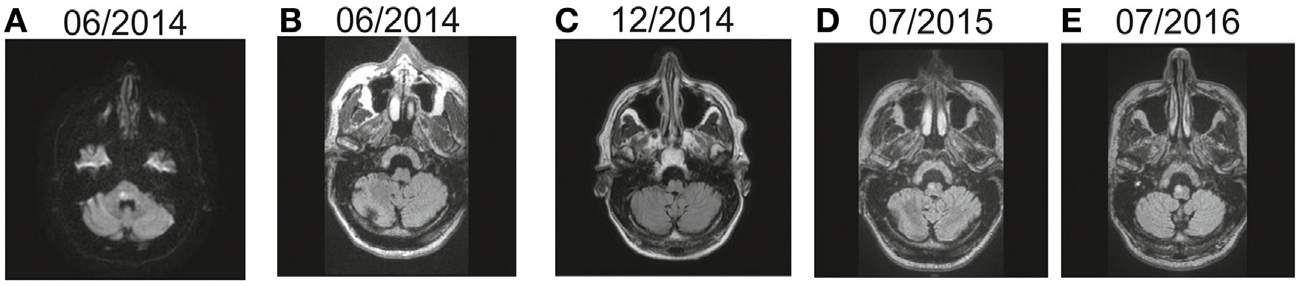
Temporal evolution of right-sided inferior olive hypersignal in a patient with symptomatic oculopalatal tremor. The patient presented a right-sided pontine tegmental lesion in June 2014 seen on the diffusion MRI scan (A), and the medulla showed no abnormal hypersignal on FLAIR MRI (B). Subsequently, right inferior olive hypersignal was observed 6 months later (C), with increasing signal 1 year later (D) and right inferior olive hypertrophy was observed 2 years later (E).
